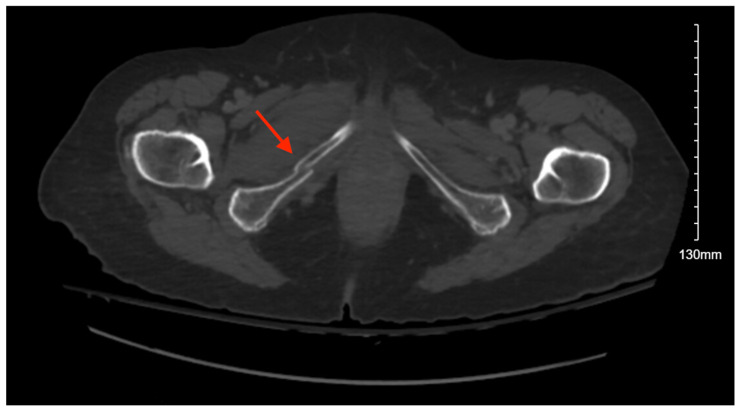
CT abdomen/pelvis images of the described fractures in case 12. Red arrows are used to indicate the location of pelvic fractures for easier identification.
